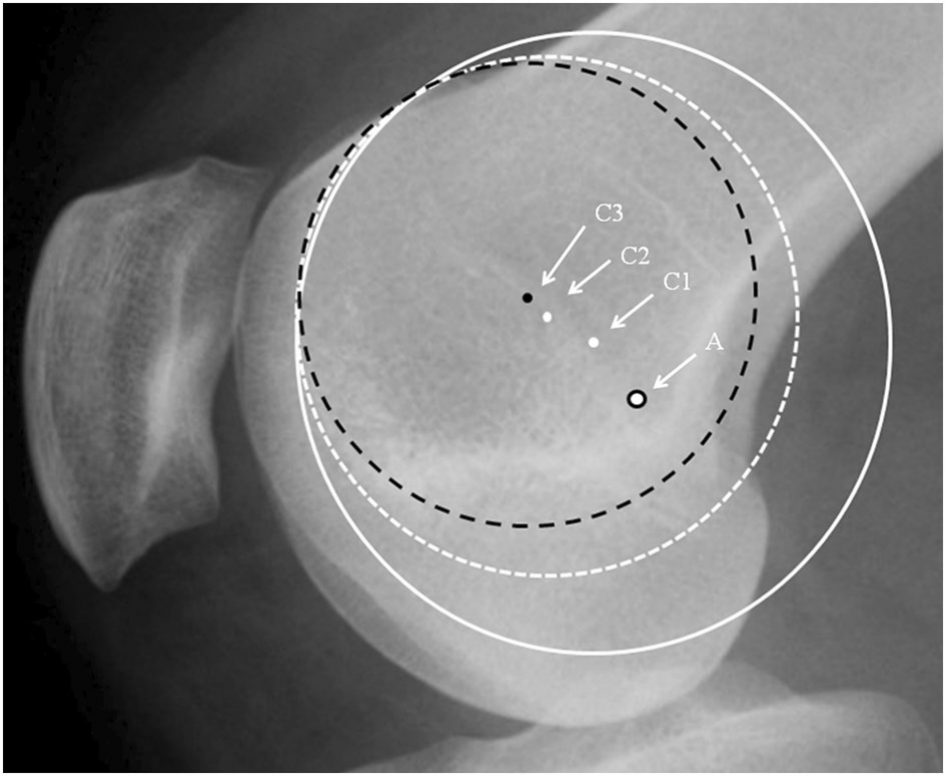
Lateral radiograph of the knee showing different circles depending on how the observer chooses the best circle-fit to the dysplastic trochlea curve: Schöttle’s point: black rimmed spot (A). Centres of white circles: white spot (C1) solid circle and (C2) dashed circle. Centre of black circle: black spot (C3)
